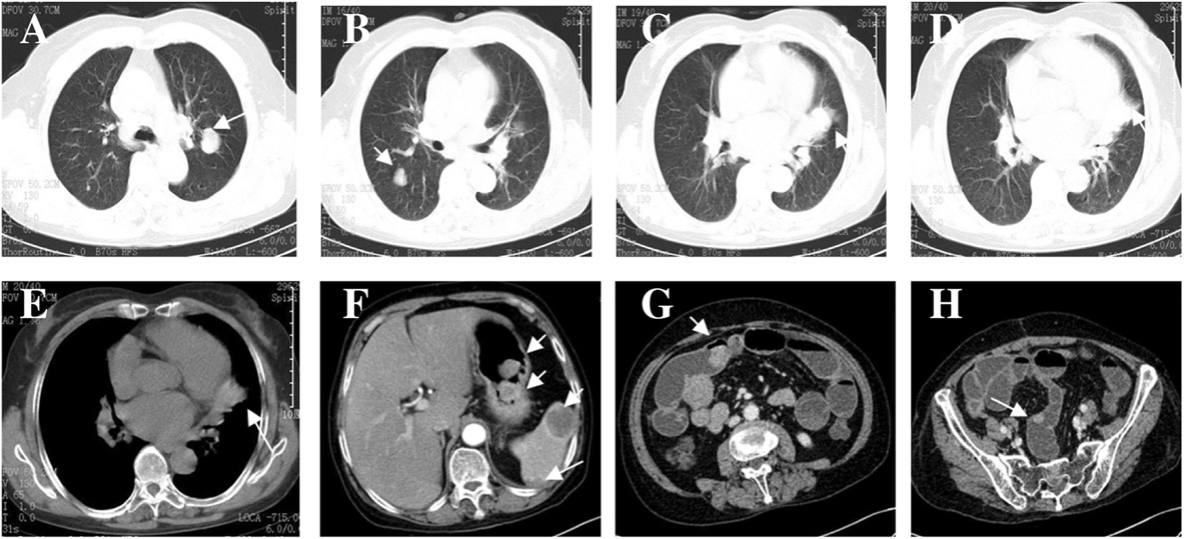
CT scans. (A, B) multiple mass in bilateral lungs; (C–E) the lobulated glomangiosarcoma was abutting the left cardiac border in the left inferior lobe; (F–H) multiple metastasis: to stomach and spleen (F), to jejunum (G), to ileum (H) (arrows).
